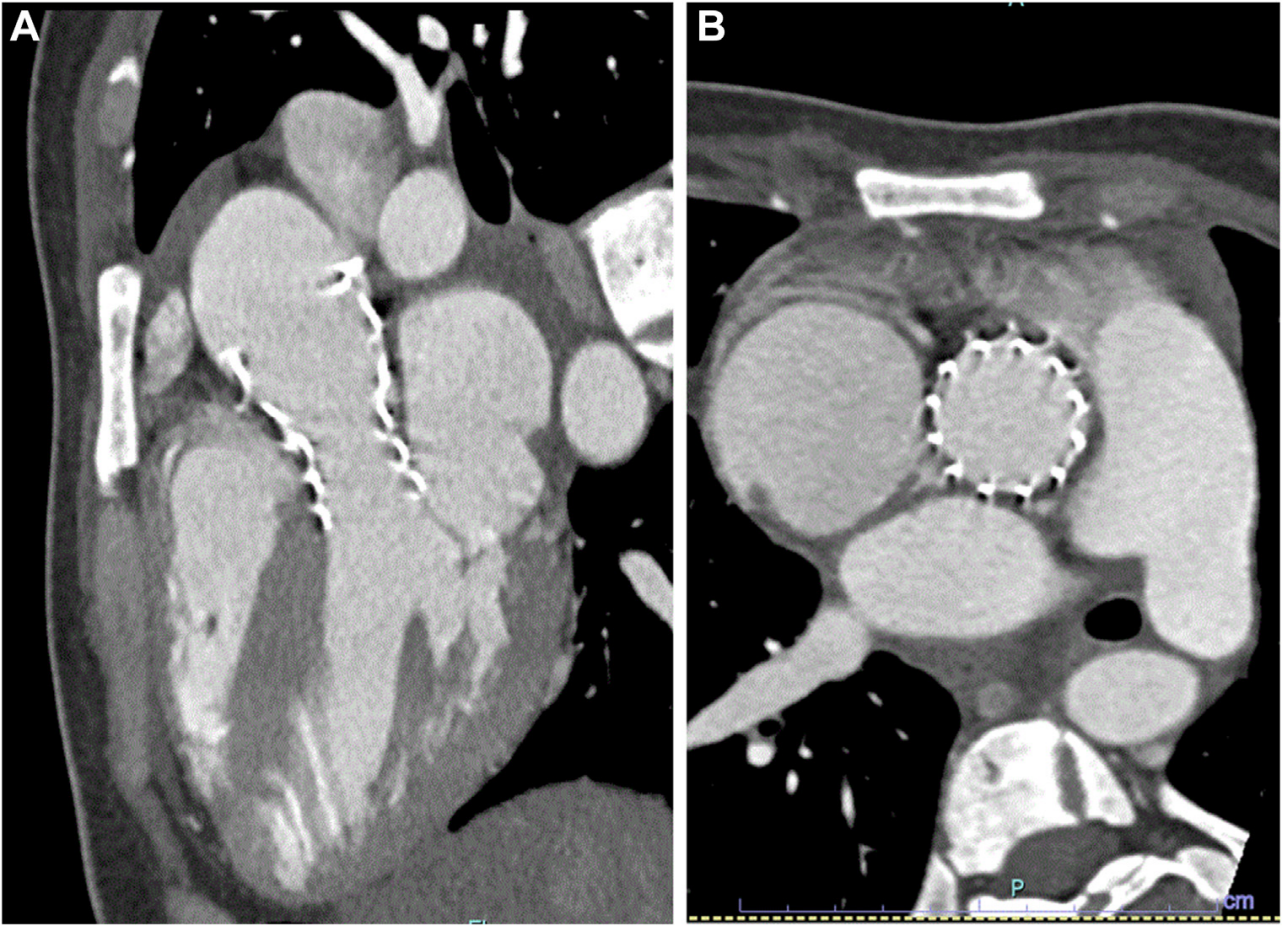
Computed Tomography 6 Months Post-Transcatheter Aortic Valve Replacement: Aortic Valve (A) Long-axis view. (B) Short-axis view.
